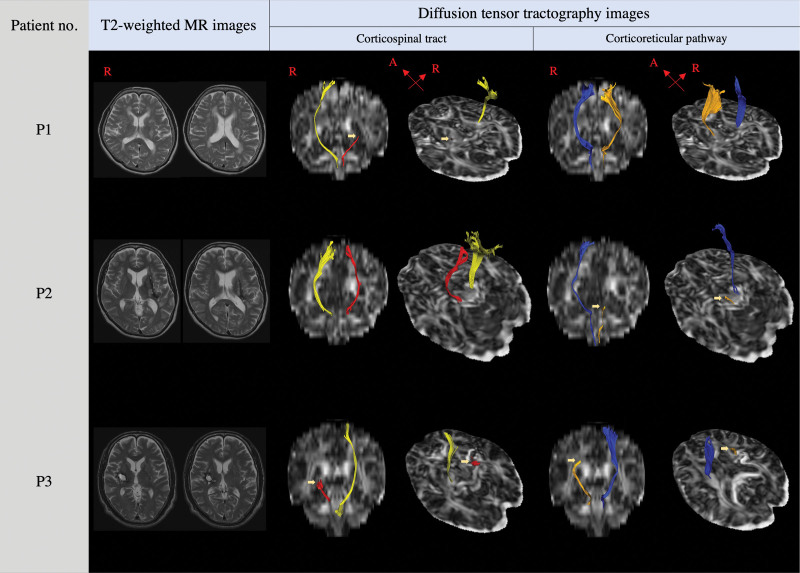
T2-weighted brain MR images (A) and diffusion tensor tractography image (B) of the CST and CRP for the 3 patients (*arrow:* discontinuation of a neural tract). CRP = corticoreticular pathway, CST = corticospinal tract.
